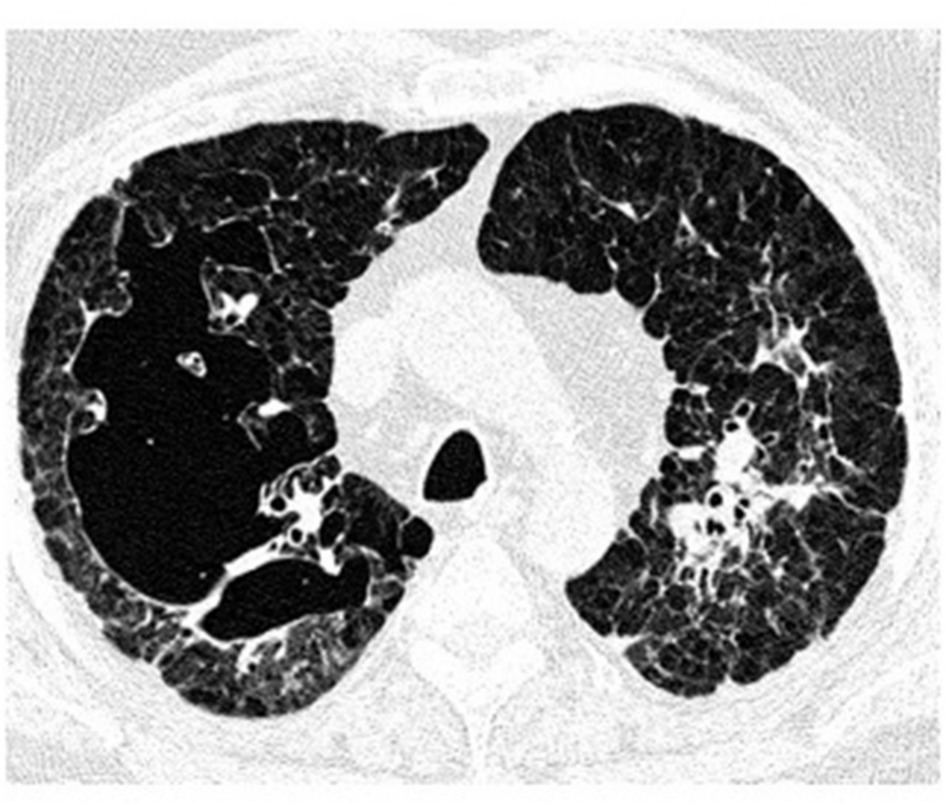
High-resolution computed tomography (HRCT) scan of a pulmonary Langerhans cell histiocytosis (PLCH) patient. Large confluent cystic lesions in both lungs.
